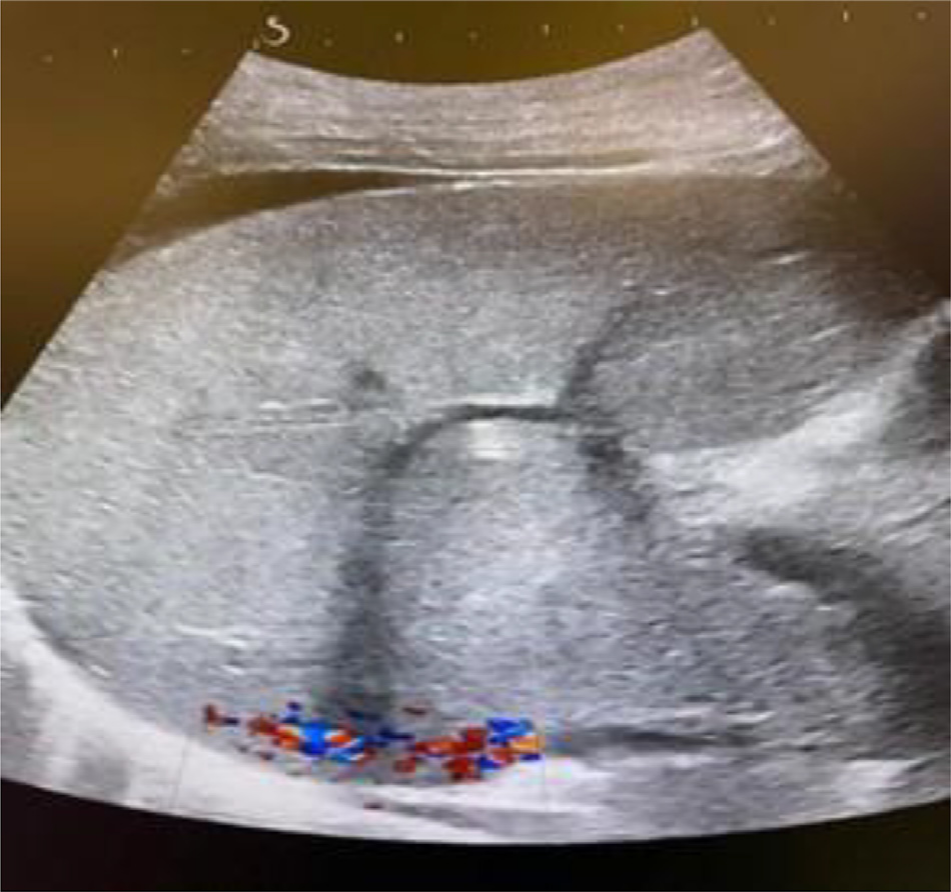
Ultrasound: Increased hepatic echogenicity. Poor visualization of hepatic veins. Abnormal Doppler flow, suggestive of hepatic vein obstruction
